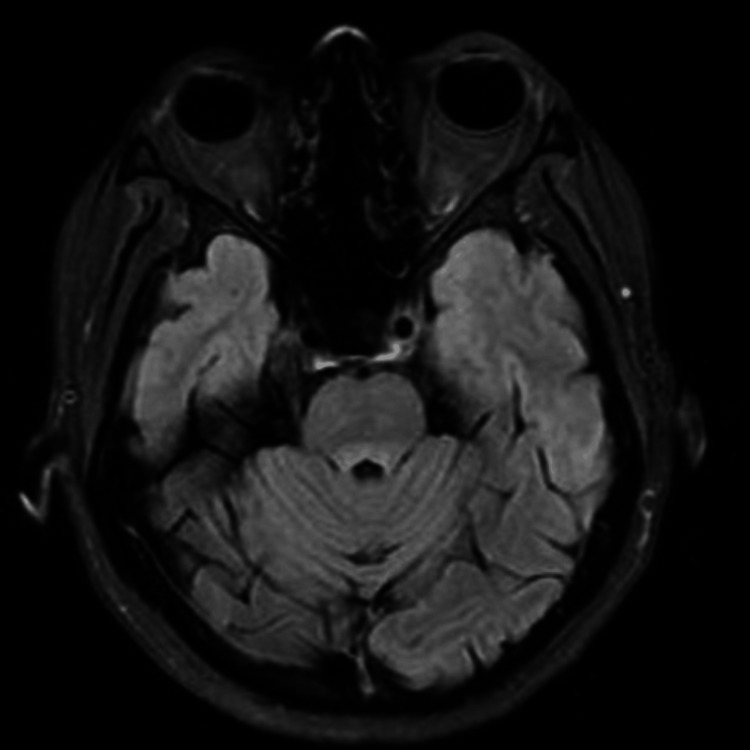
T2 FLAIR study of brain MRI showing the resolving signal abnormality in the dorsal pons MRI: magnetic resonance imaging; FLAIR: fluid-attenuated inversion recovery
